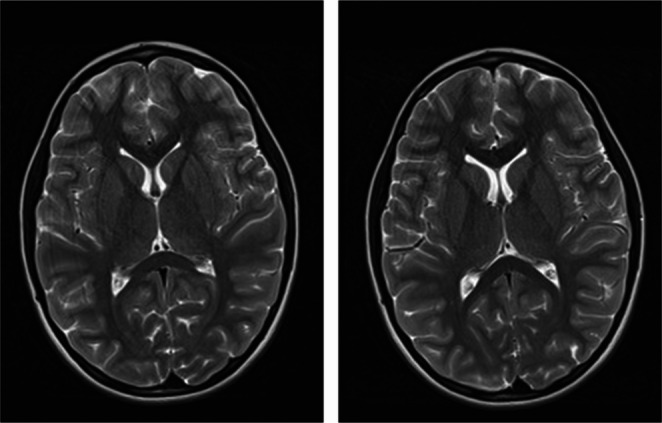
Brain MRI image. Regression of observed lesions. Solitary small focus found in the posterior region of the left putamen
